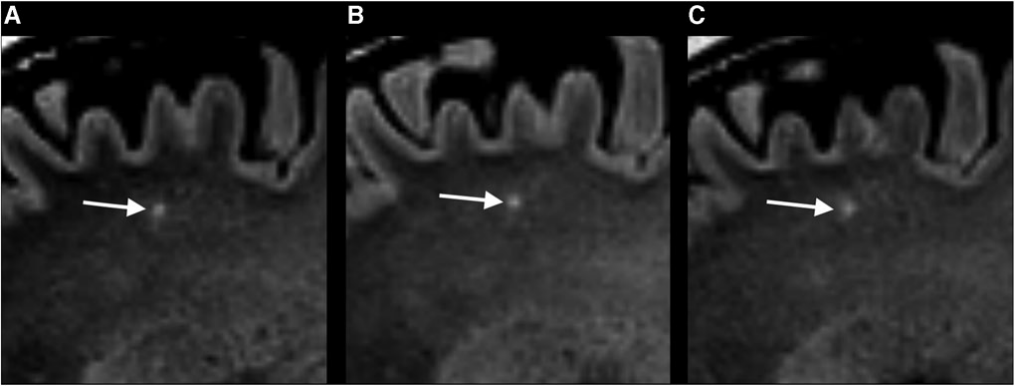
(A–C) A non-traumatic white-matter hyperintensity (WMH) on fluid attenuated inversion recovery (FLAIR) scans in the sagittal plane of the same patient at three different time points. (A) The very early FLAIR scan showed a hyperintense lesion in the deep gray matter in the left frontal lobe (arrow) that persisted at the (B) 3 month and (C) 12 month examination. The lesion was not present at the susceptibility weighted image scan at any time point.
